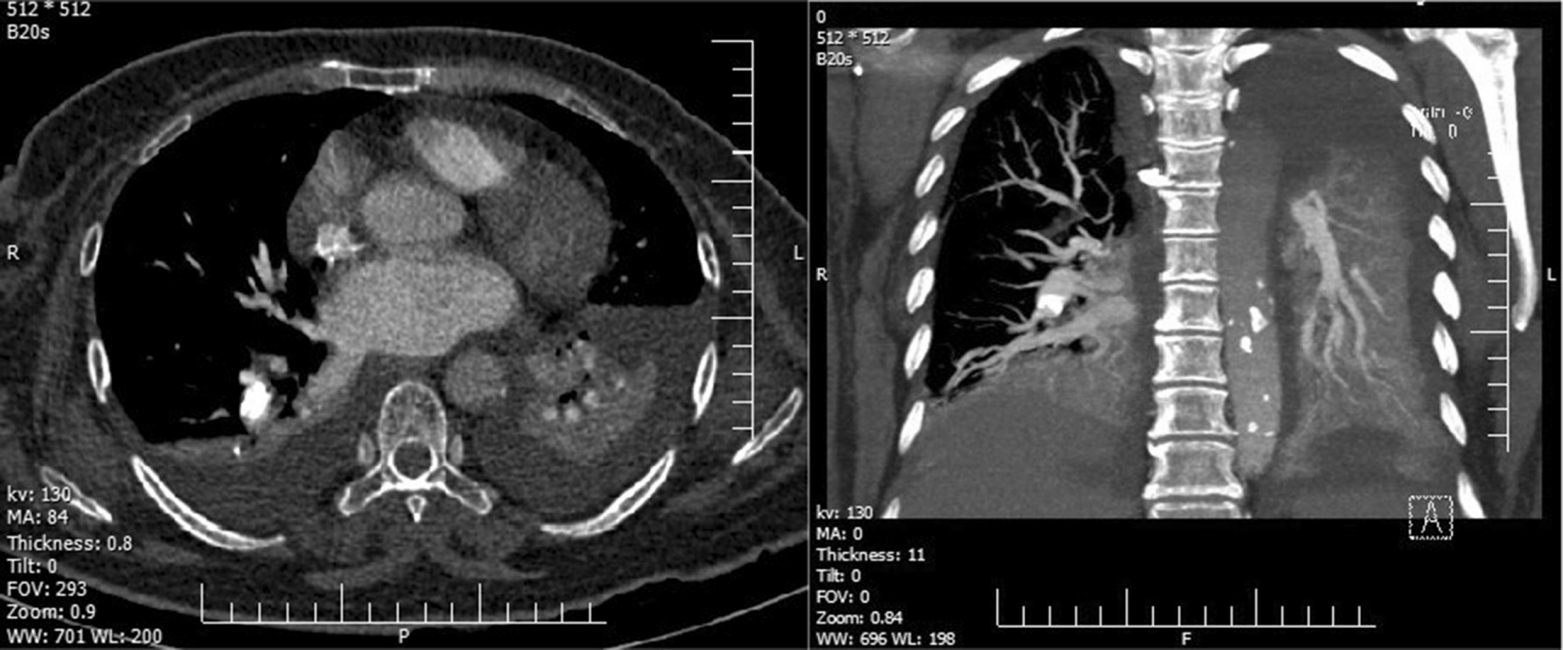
The spiral chest computed tomography angiography (a: axial; b: coronal maximum intensity projection) shows a hyperdense filling defect in the posterior basal branch of the right pulmonary artery, consistent with cement embolization.
